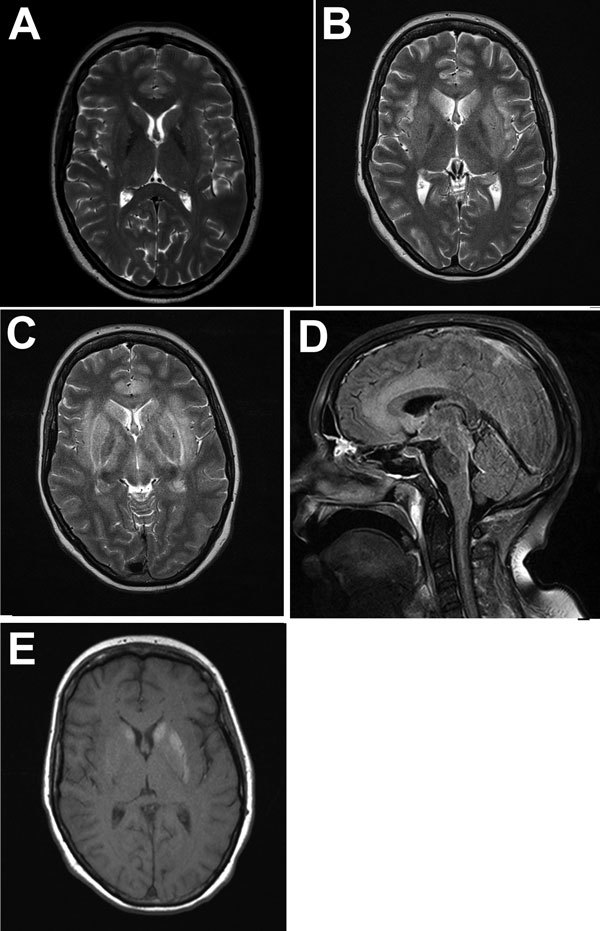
Magnetic resonance imaging of the brain throughout the course of the disease in patient who died of limbic encephalitis caused by variegated squirrel bornavirus 1 (VSBV-1), Germany, 2013. A) T2-weighted transversal image at admission showing no pathologic changes. B) T2-weighted image 3 weeks after admission showing edema in limbic structures (insula, hippocampus, anterior cingulate) and in the basal ganglia. C) T2-weighted image 4 weeks after admission showing progressive edema. Additional myelopathy extended from the medulla down into the thoracic segments (not shown). D) FLAIR image 4 weeks after admission showing edema in the anterior cingulate cortex. E) T1-weighted image 4 weeks after admission without contrast showing slight hemorrhage in the basal ganglia.
